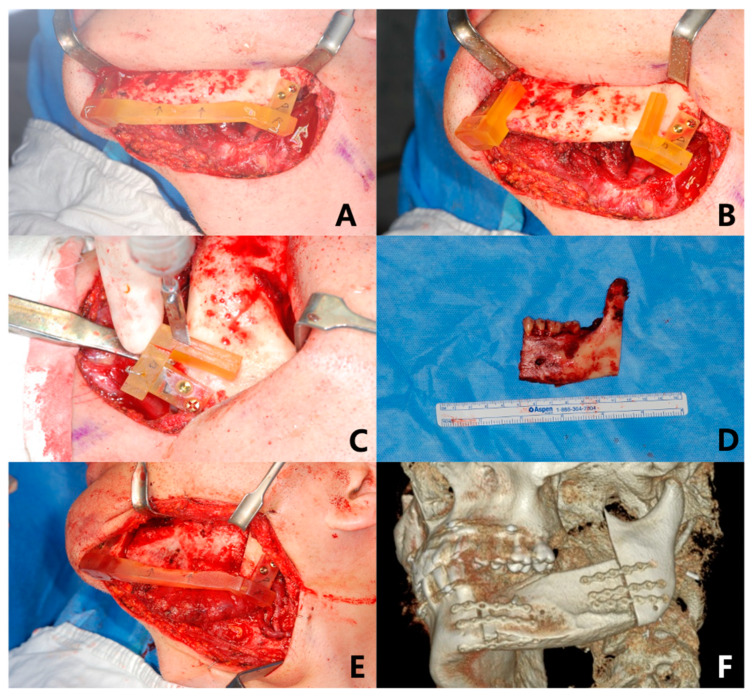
Mandibular reconstruction assisted by 3D surgical guide for case of deep circumflex iliac artery free flap (DCIA). (A) Mandibular positioning guide was temporarily fixed before mandibulectomy. (B) Cutting guide was engaged to cut mandible as planned on 3D virtual surgery after removal of positioning guide. (C) Sawing was performed according to cutting guide. (D) Body of mandible with tumor was removed. (E) Positioning guide was repositioned, and DCIA was set and fixed while original occlusion and condyle position were maintained. (F) Flap position was assessed by postoperative CT.
